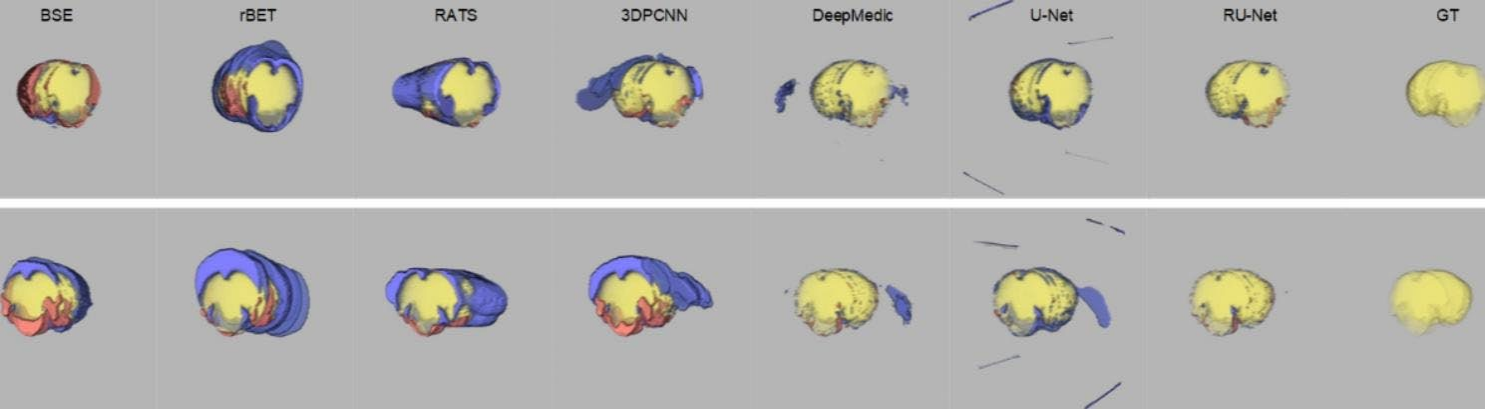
Visual comparison of DWI skull stripping results in 3-D view using different methods. Blue: $${\theta }_{FP}$$. Red: $${\theta }_{FN}$$. Top row: Subject 16. Bottom row: Subject 40
